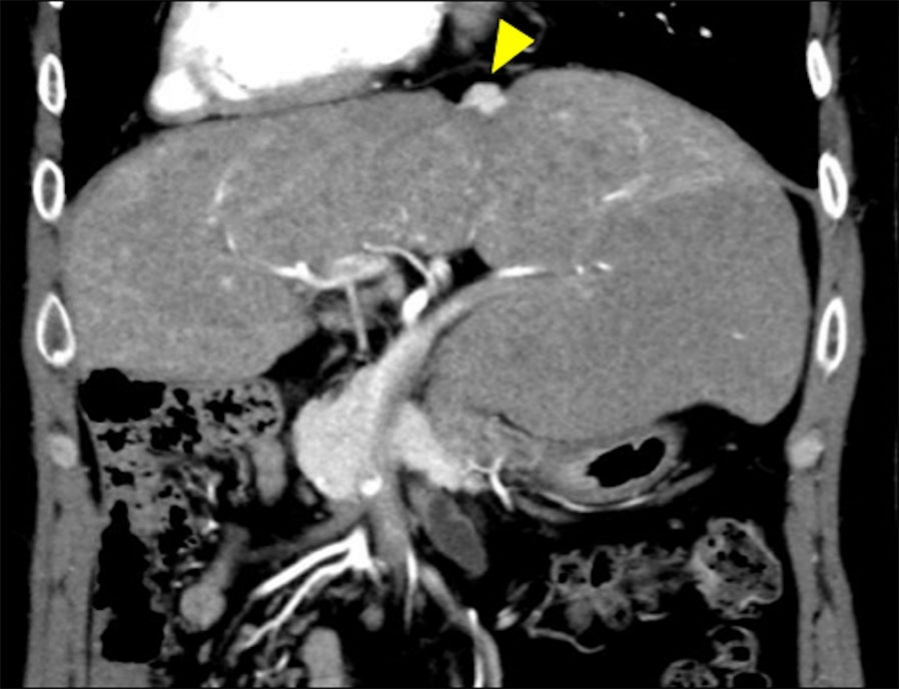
Abdominal dynamic contrast-enhanced CT (arterial phase). Arrowhead shows the tumor protruding from the liver surface
